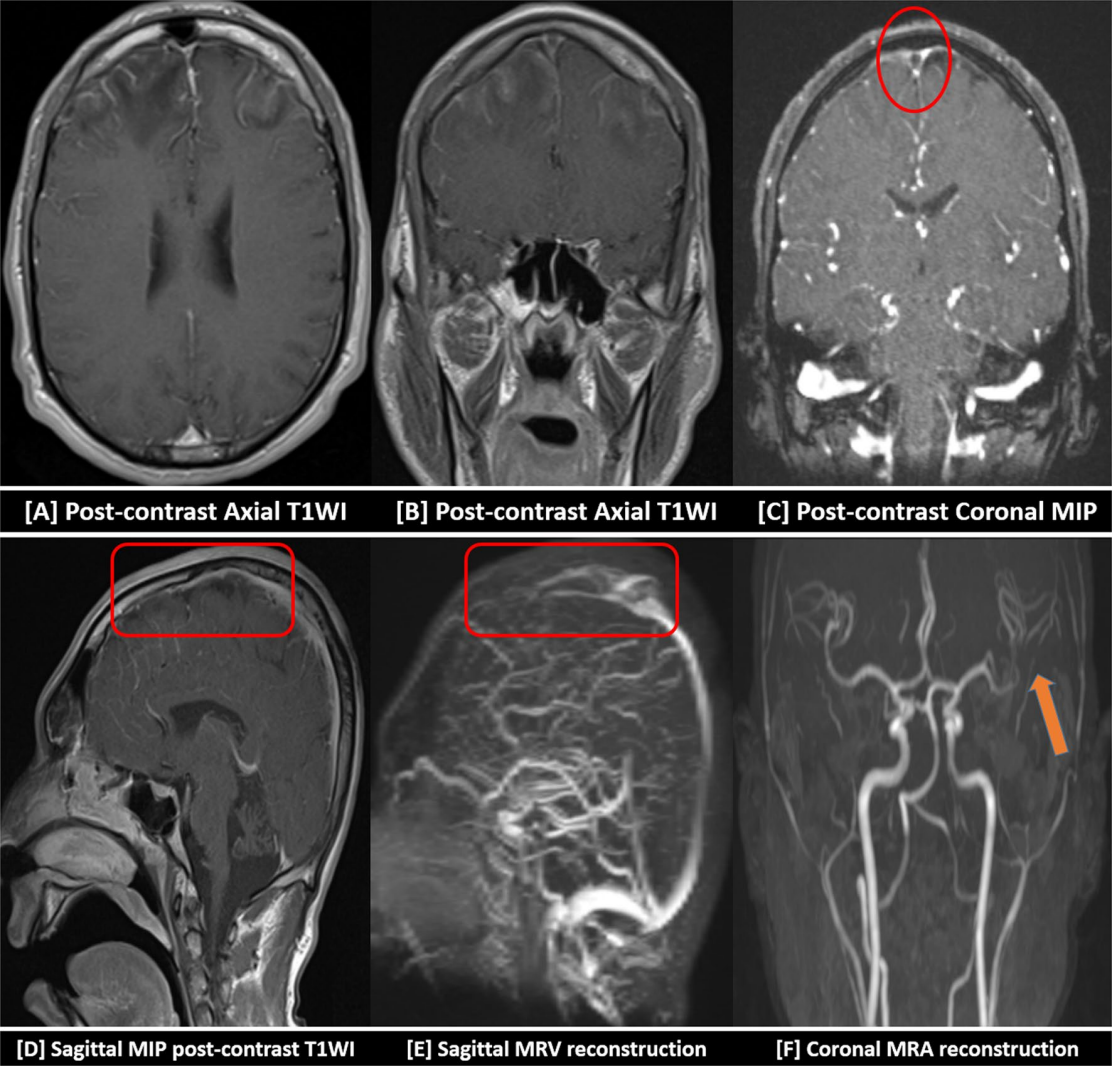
Contrast-enhanced MRI with MRV and MRA were performed for the above-mentioned patient (Fig. 6). [A, B] Patchy ischemic gyral enhancement was noted after IV contrast administration in T1-WI. [C] Central filling defect (delta sign) was noted in the superior sagittal sinus at the post-contrast coronal T1-WI with MIP reconstruction (red circle). [D] Long segment filling defect was seen at the post-contrast sagittal T1-WI with MIP reconstruction (red square). [E] This was also demonstrated in sagittal MRV reconstruction (red square). [F] MRA revealed attenuation of the lumen of the left M3 at the left Sylvian fissure as well as distal M4 cortical branches (orange arrow) … Final diagnosis was bilateral frontoparietal infarctions sequel to superior sagittal sinus thrombosis with hyper-acute hemorrhagic transformation. Additionally, left M3 and M4 attenuation was reported
